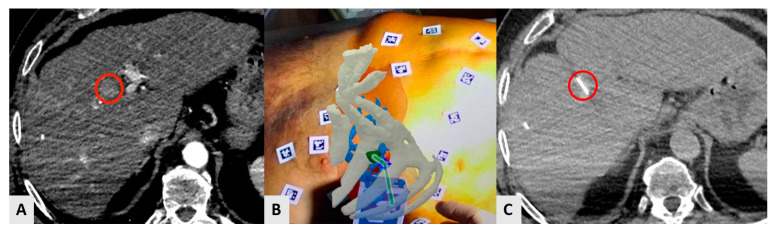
A 73-year-old male HCC patient was treated with percutaneous radiofrequency ablation in segment VIII. The ablation procedure was performed under CT guidance using an AR navigation device. (A) The small HCC (red circle) is visible in arterial phase. (B) AR guidance from the physician’s point of view. The liver is in red; the bones of thoracic case are in white; the liver vessels are in light-blue, and the lesion is in green. The green line shows the trajectory that connects the tip of the needle to the center of the target in real time. (C) Axial CT scan showing the tip of the needle (red circle) precisely located in the center of the lesion after the guidance by AR.
